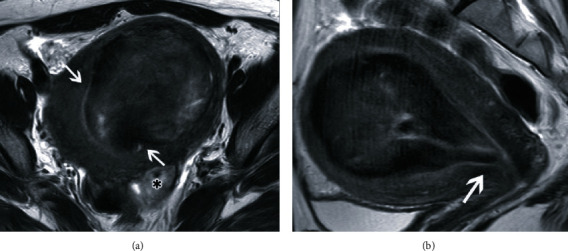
Magnetic resonance images 1 month after uterine artery embolization. (a) T2-weighted axial image demonstrates the fibroid protruding from the endometrium into the endometrial cavity (white arrows). The asterisk (∗) shows another degenerative uterine fibroid. (b) T2-weighted sagittal image demonstrates prolapse of the fibroid into the internal os (white arrow). A small portion of the fibroid is protruding into the uterine cavity.
